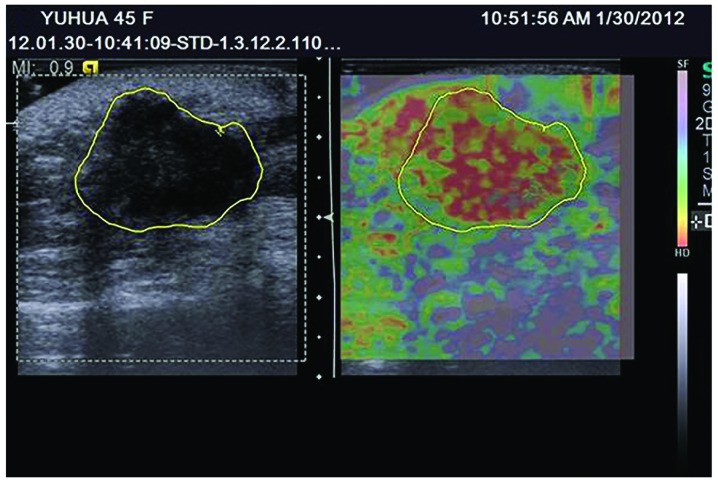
Surgical pathology in a 45-year-old woman with a palpable mass in the outer upper quadrant of her right breast proved an invasive ductal carcinoma of the breast. Left image: Two dimensional sonogram. Right image: Elastography map, 5 points.
